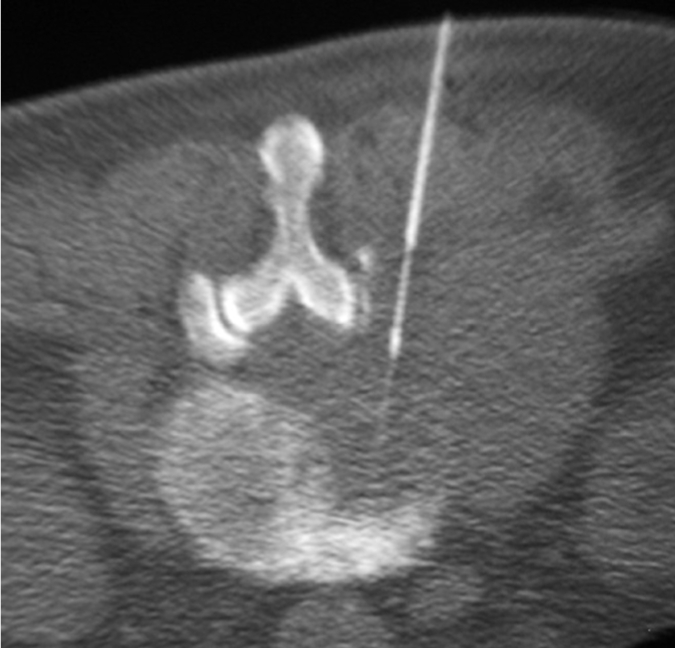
Use of a Temno cutting needle (Bauer Medical International, Santo Domingo, Dominican Republic) for biopsy of a large L4 vertebral lesion in a 79-year-old man with hepatocellular carcinoma. CT fluoroscopic image taken with the patient lying prone shows the tip of the cutting needle within the soft tissue component of the destructive osteolytic lesion that has extended into the right psoas muscle. Note that the bevelled-point stylet has been advanced in preparation for firing of the cutting cannula.
